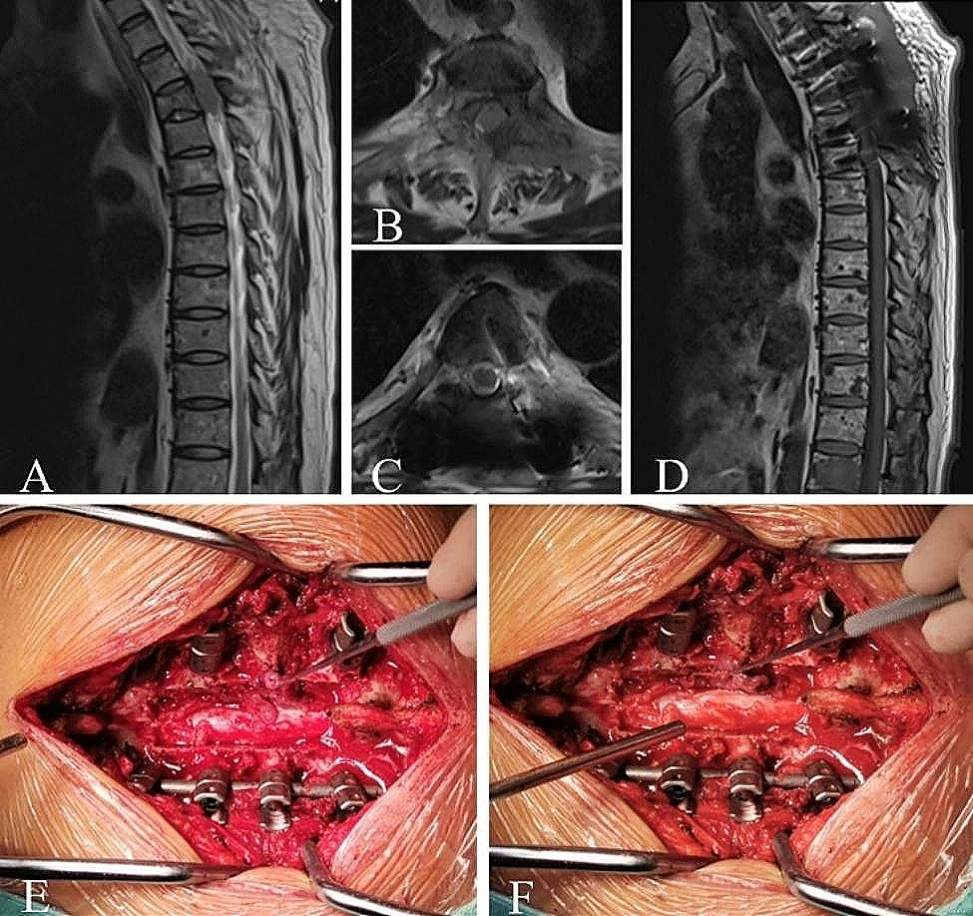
Spinal cord compression due to plasmacytoma. (A-B) Preoperative MRI shows a tumor compressing the spinal cord at the T2-4 levels, with epidural spinal cord compression graded as level 3. (C-D) Postoperative MRI reveals cerebrospinal fluid filling around the spinal cord without obvious compression, with pedicle screws well-placed and spinal sequences stable. (E-F) Intraoperatively, fish-like tumor tissue is visible, and laminectomy and tumor resection are performed to decompress the spinal cord
